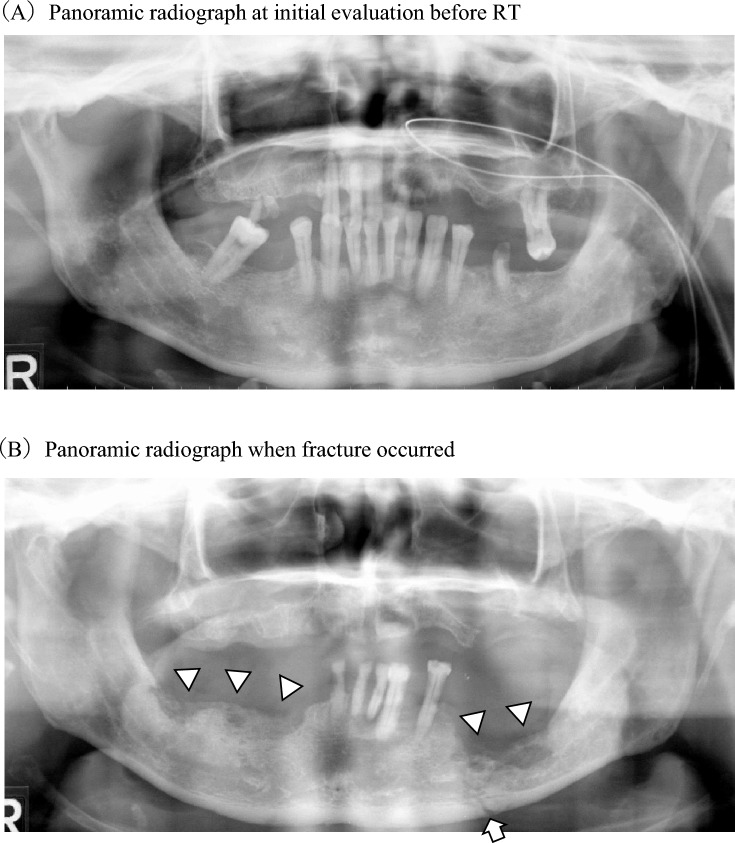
Panoramic radiograph. (A) Panoramic radiograph at initial evaluation before RT. (B) Panoramic radiograph when fracture occurred. Triangles represent the site of ORN (▽) and arrows represent the site of pathological fracture (→).
